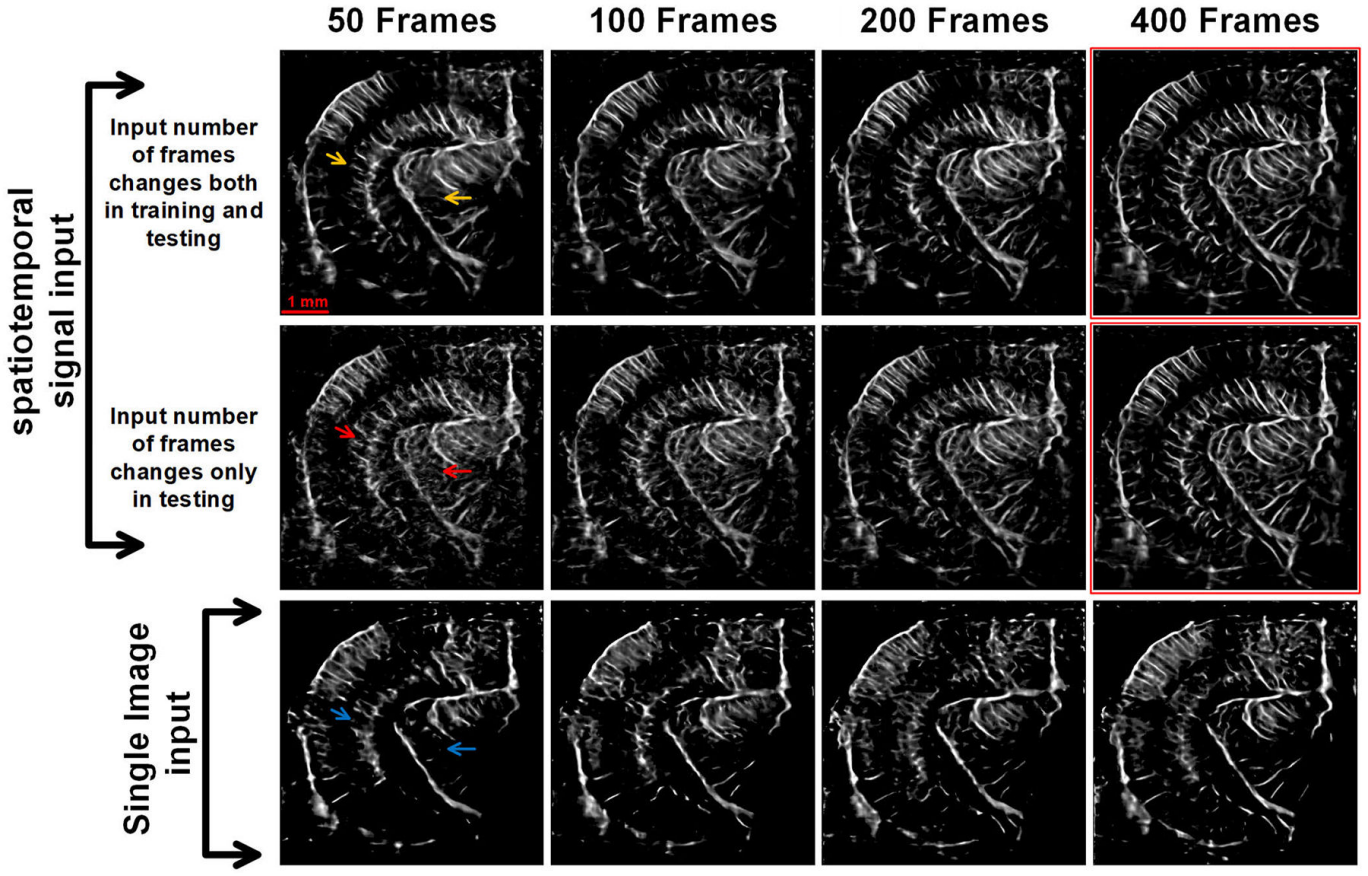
CS-PD using different ways to input ultrasound signal: input number of frames changing both in the network training and testing (first row), input number of frames changing only in the network testing (second row), and inputting power Doppler image after accumulating the spatiotemporal ultrasound signal (third row).
